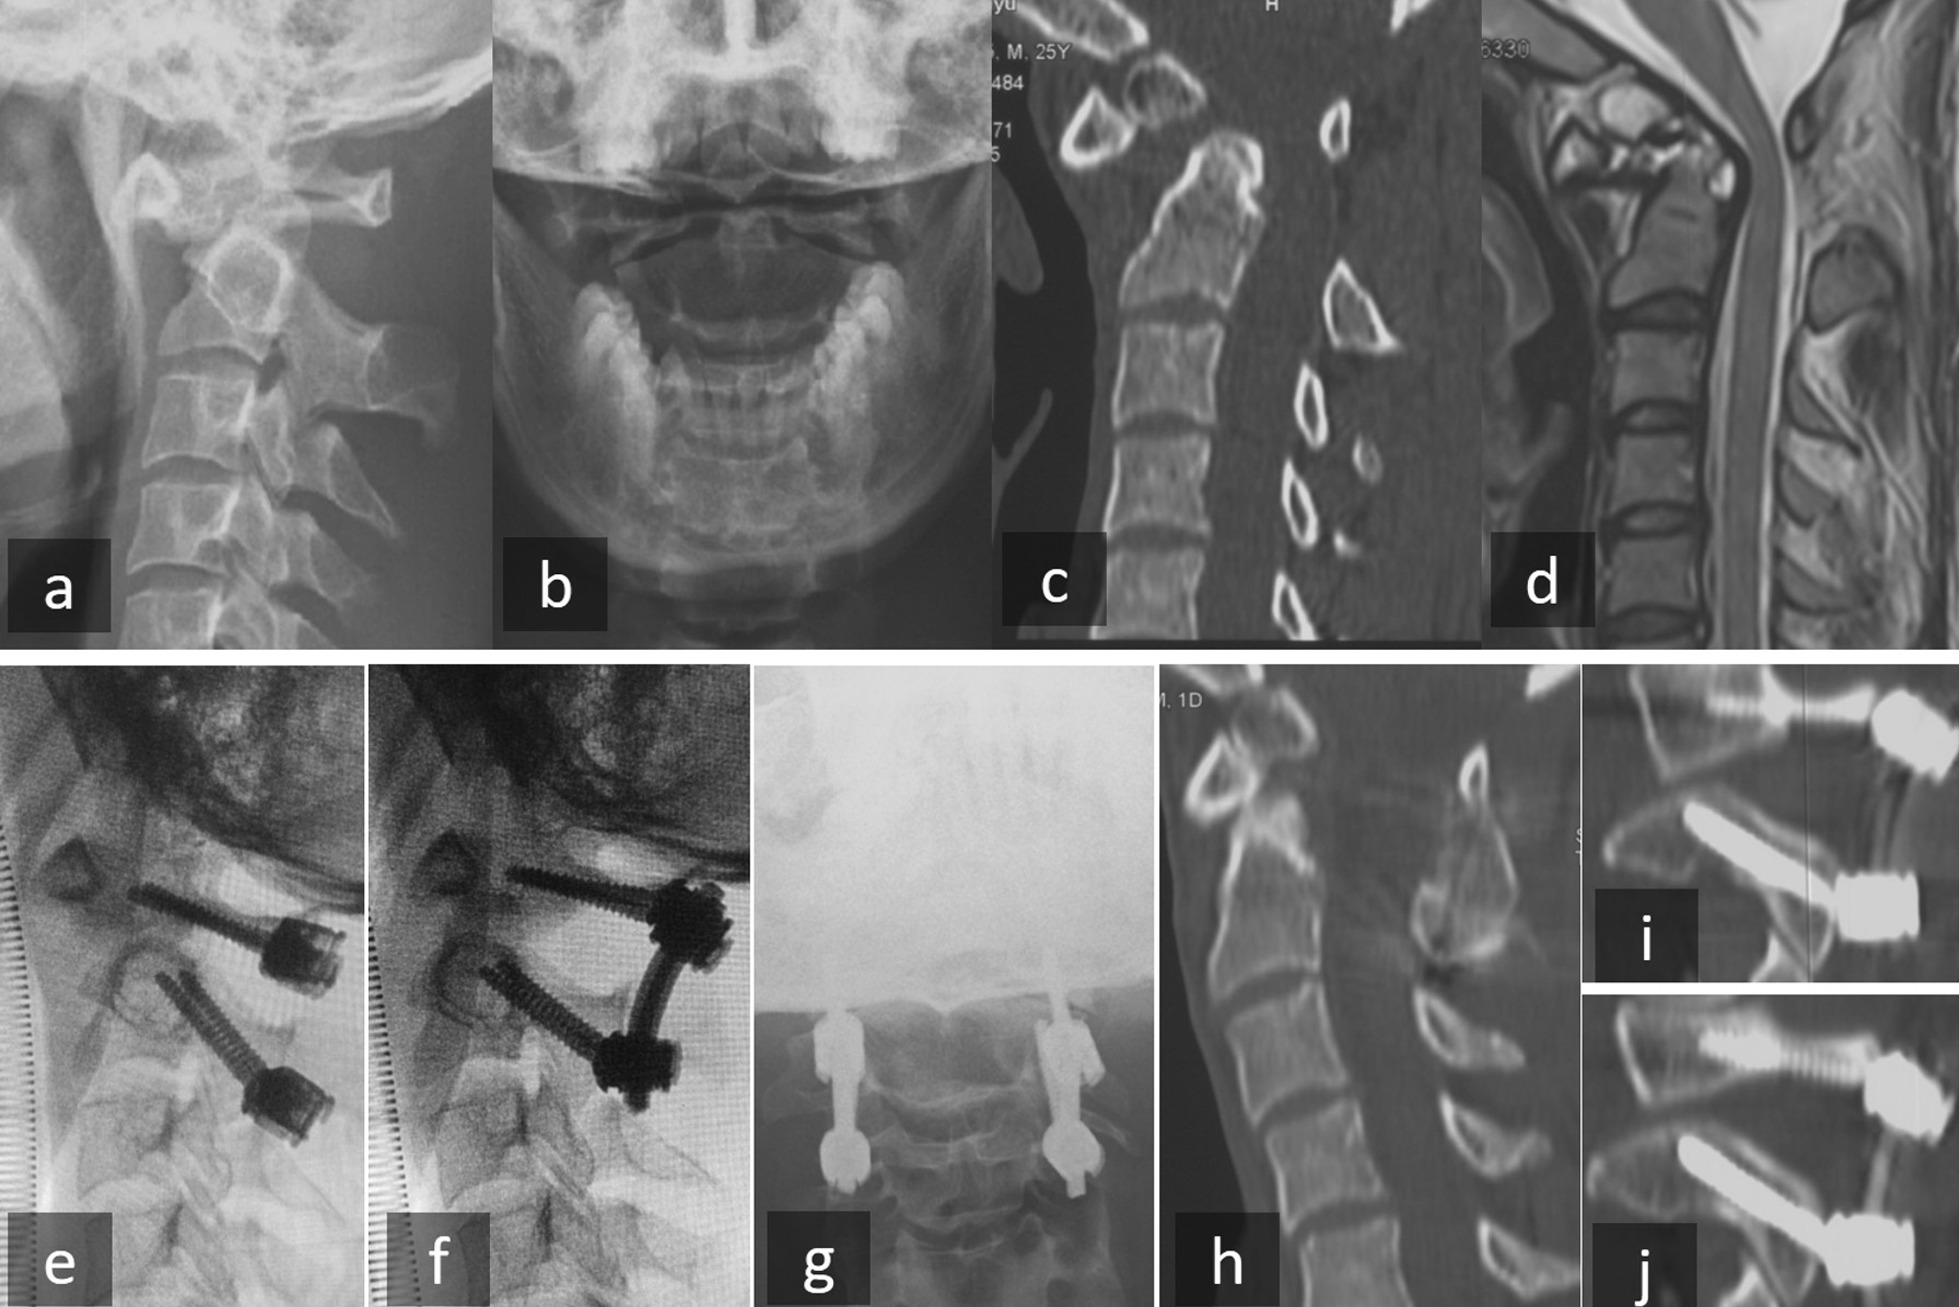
A 24-year-old man with Os odontoideum. a–c Preoperative X-ray and CT scan showed Os odontoideum and atlantoaxial dislocation. d Preoperative MRI showed spinal cord compression. e Intraoperative X-ray showed the position of C1 PS combined with modified C2 isthmus screw and f reduction. g Postoperative anterior–posterior X-ray, and h–j Postoperative CT scan showed good reduction and position of modified C2 isthmus screw. *PS* pedicle screw
